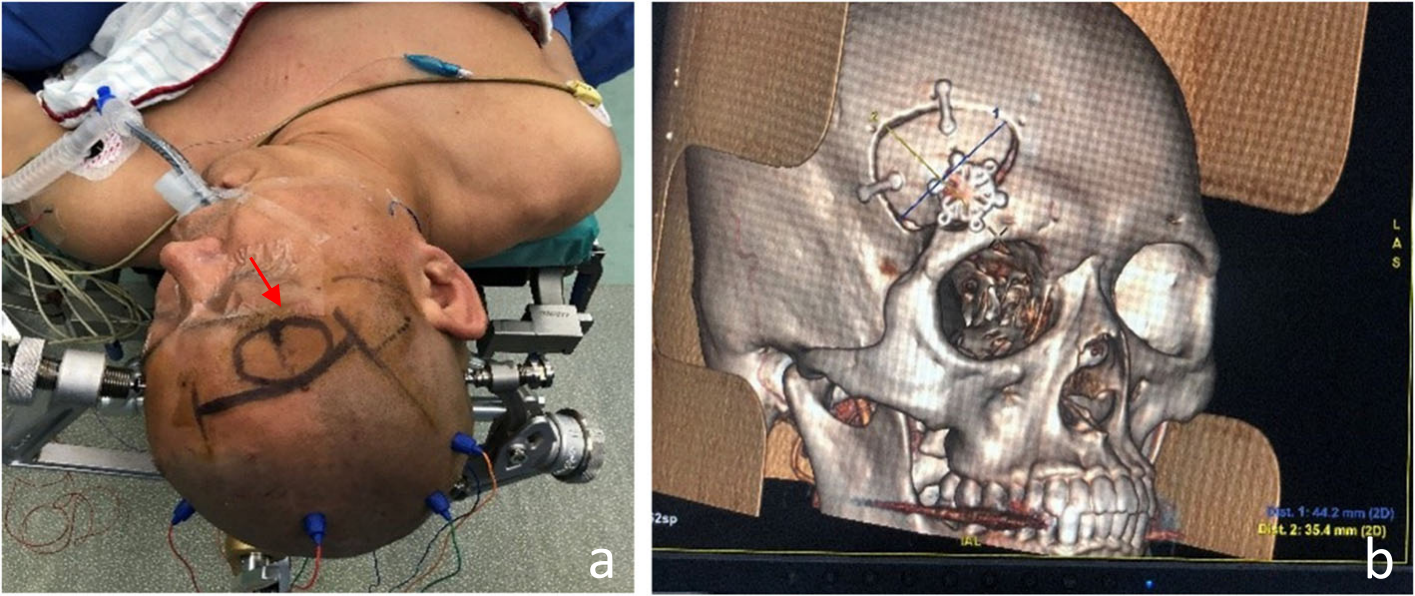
Preoperative preparation and postoperative bone flap display of ruptured anterior communicating aneurysm by reconstruction clipping via supraorbital lateral keyhole approach. a The patient had a ruptured anterior communicating artery aneurysm. The patient’s head was slightly left to the upper three-point skull frame, which was connected with electrophysiological monitoring. The arrow showed the approximate range of bone flap. b Postoperative 3D-CTA showed that the bone flap was well fixed
